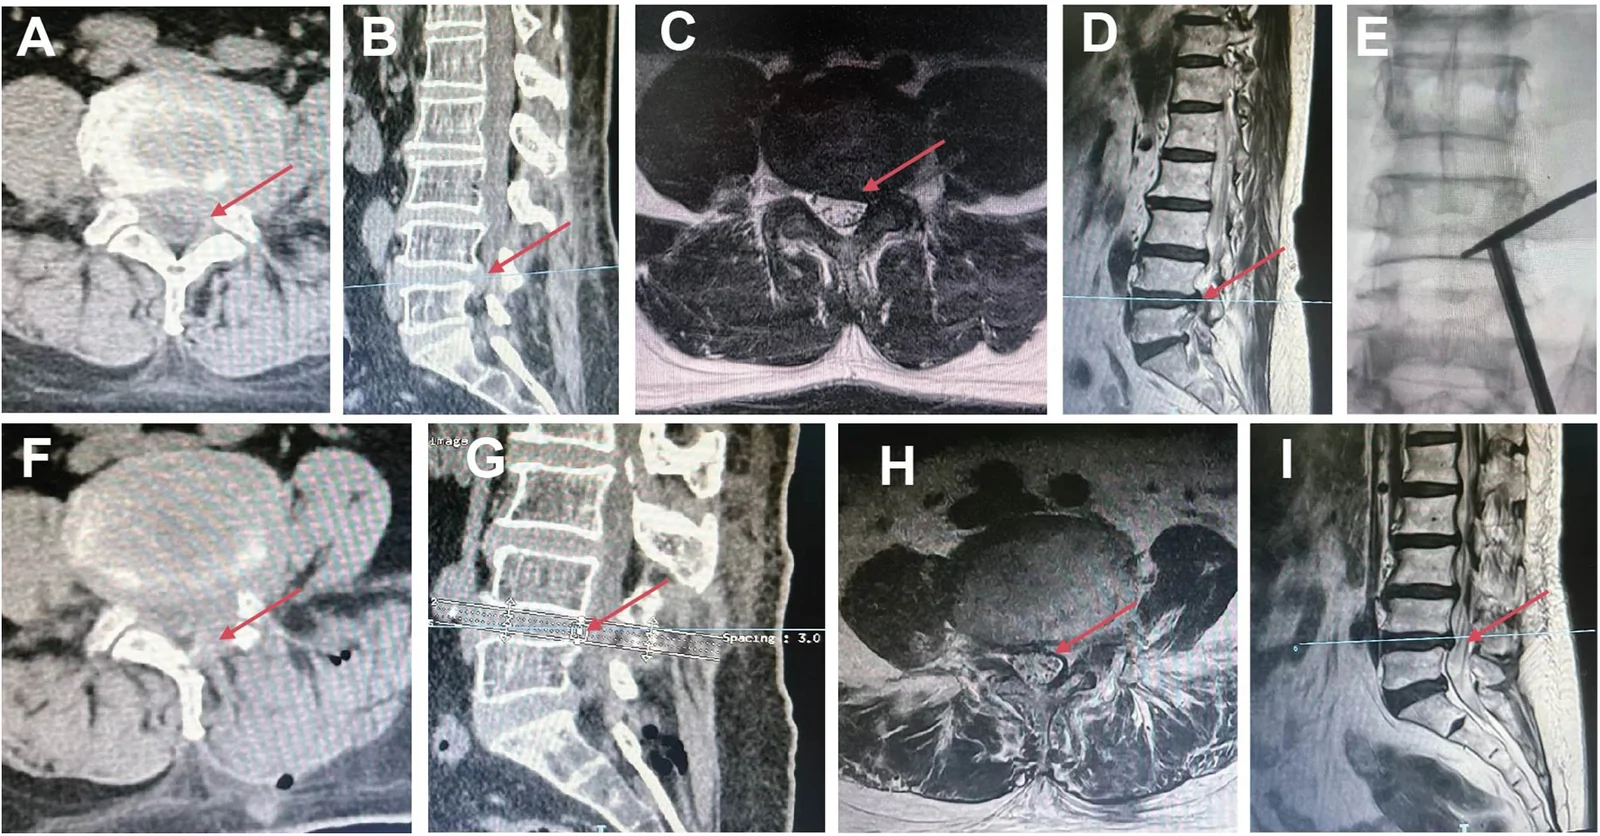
Preoperative and postoperative lumbar imaging sequences of representative single-segment lumbar disc herniation patients. The red arrows in all subgraphs mark the target herniated disc segment and surgical repair area. (A,B) Axial and sagittal preoperative lumbar CT demonstrating intervertebral disc protrusion; (C,D) Axial and sagittal preoperative T2-weighted MRI confirming the herniated mass compressing the neural canal; (E) Intraoperative fluoroscopic localization radiograph for the target intervertebral space; (F,G) Postoperative axial and sagittal CT showing adequate bony decompression of the spinal canal; (H,I) Postoperative 6-month axial and sagittal T2-weighted MRI revealing continuous fibrous tissue coverage of the sutured annular defect.
